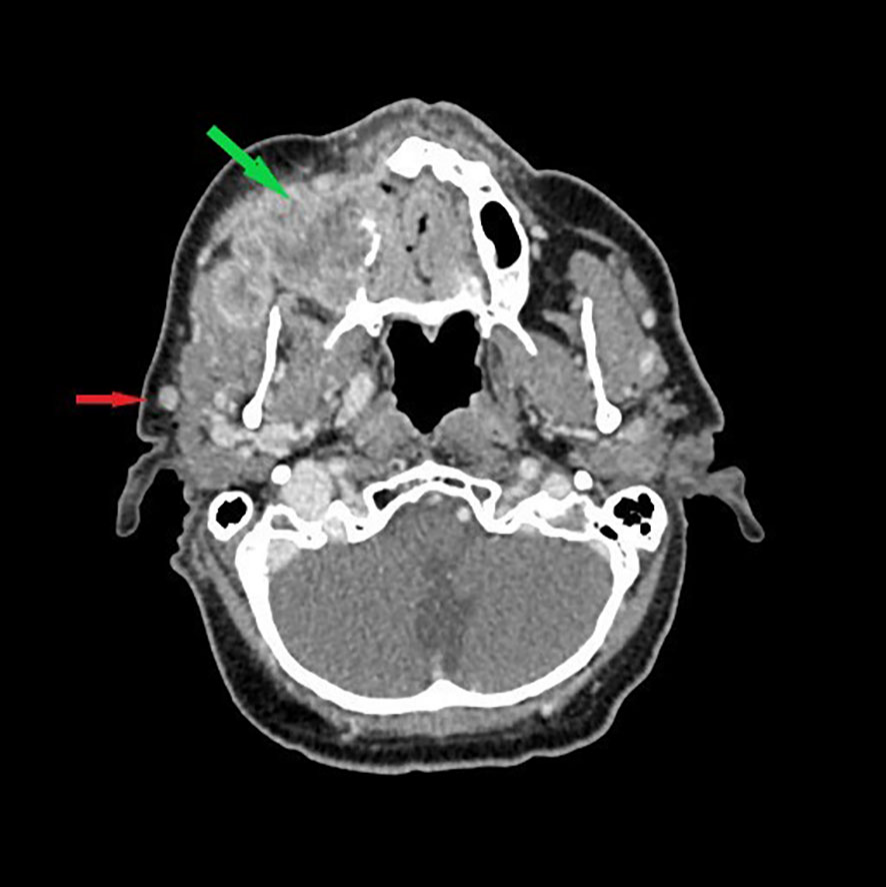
Contrast-enhanced axial view CT scan showing a 7-mm enlarged right parotid lymph node (arrow) in the patient with advanced SCC of the right maxilla (green arrow). During surgery, frozen sections of the parotid lymph node revealed no metastasis, only chronic inflammation, and parotidectomy was not performed.
